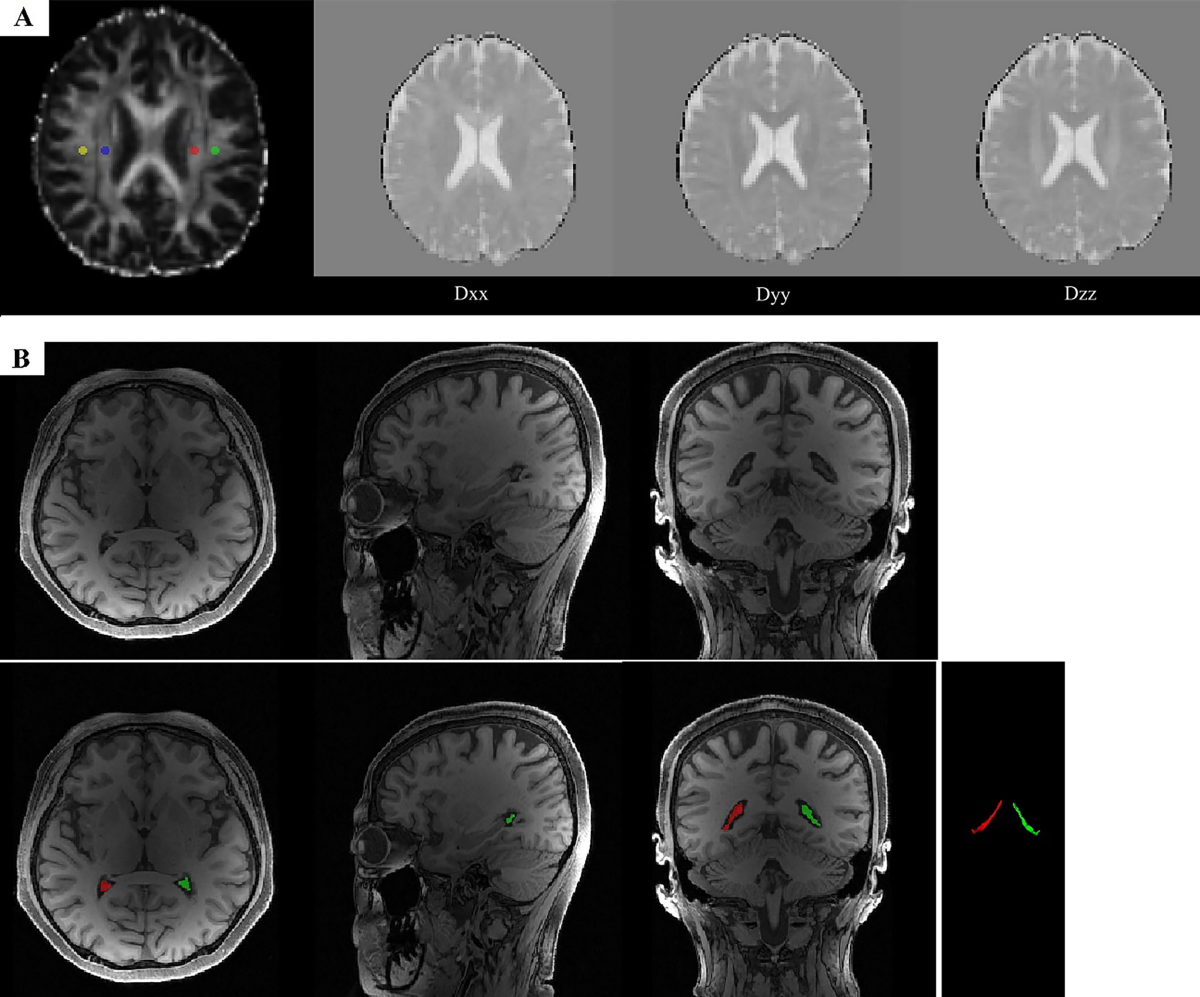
(A) Diffusion-weighted preprocessed images were used to calculate the DTI-ALPS index. Predefined ROIs for projection (superior corona radiata, yellow and green) and association (superior longitudinal fasciculus, blue and red) fibers at the level of the lateral ventricle body were used to derive the diffusivity values of Dxxproj, Dxxassoc, Dyyproj, and Dzzassoc. (B) Example of choroid plexus segmentation (before and after) on axial, sagittal, and coronal slices from a three-dimensional T1-weighted sequence.
